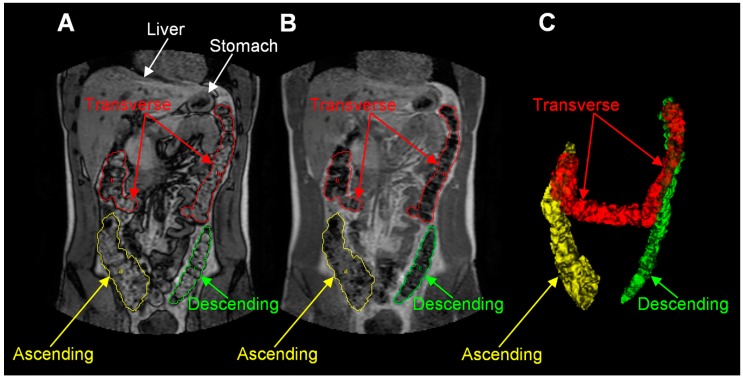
MRI images for colonic anatomy. (A,B) are different out of phase and in phase water and fat dual-echo imaging and (C) is a 3D reconstruction. Reproduced from [33], Blackwell Publishing LTD, 2013 with permission.
